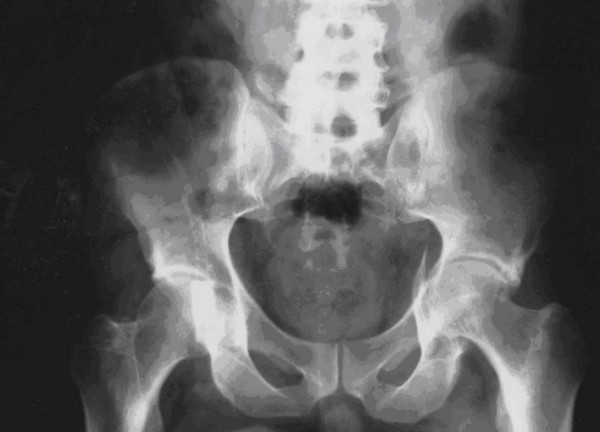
Radiography of hip after seizures showing left acetabular fracture (Case 1).
